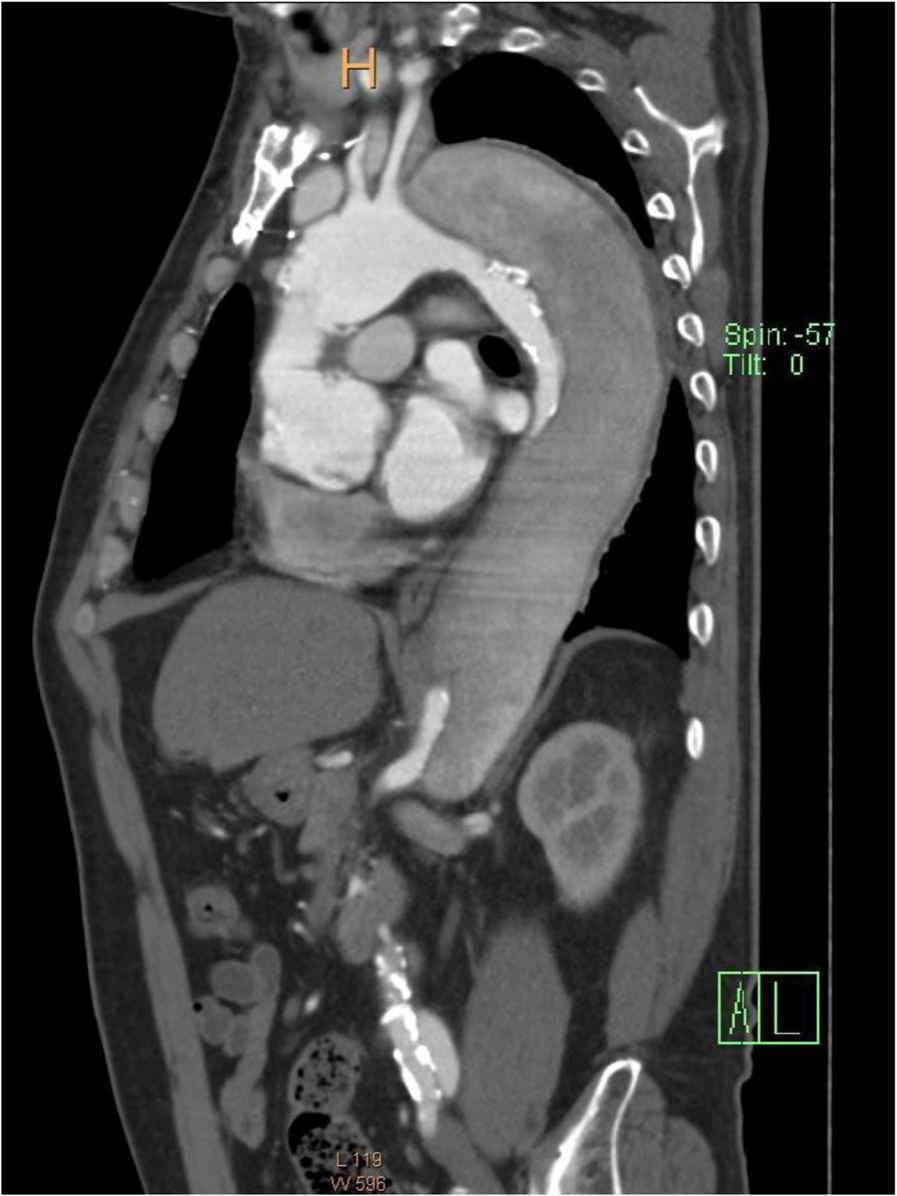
Computed tomography (CT) image showing an aneurysm of the sinus of Valsalva and an extended aortic aneurysm, spanning the proximal descending aorta to level of the abdominal aorta.
